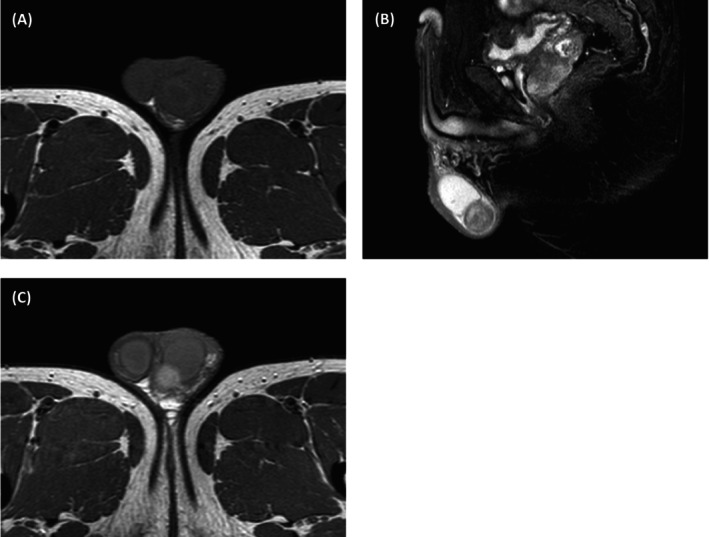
Magnetic resonance imaging of paratesticular adenomatoid tumor. (A) T1 weighted image showed the mass was slightly high signal with a patchy low signal. (B) T2 weighted image showed that the mass was high, with a patchy low signal. (C) Enhanced magnetic resonance imaging scans showed the mass was a heterogeneous enhancement.
